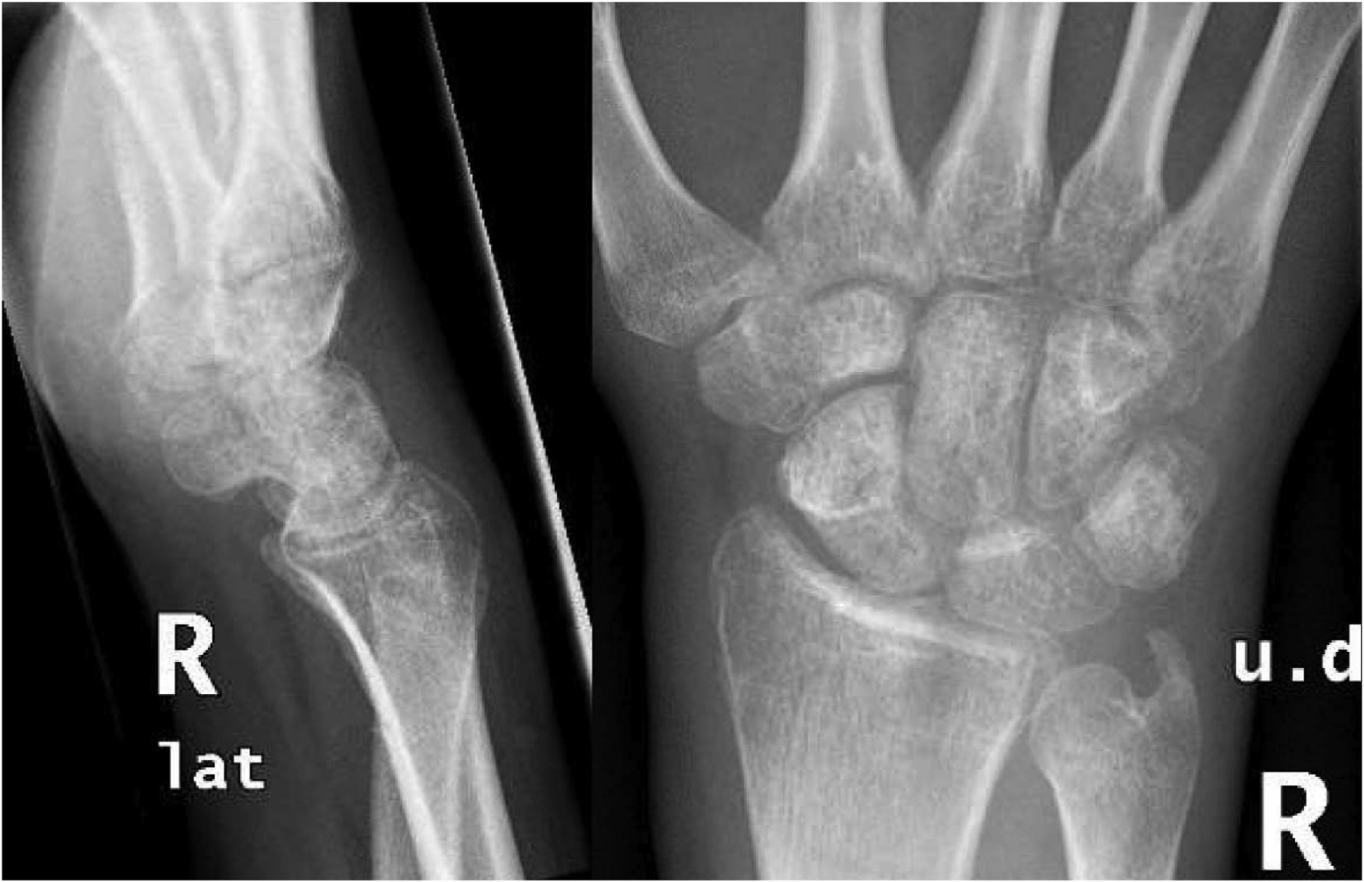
Radiographs of the wrist after Kirschner wires removal.
